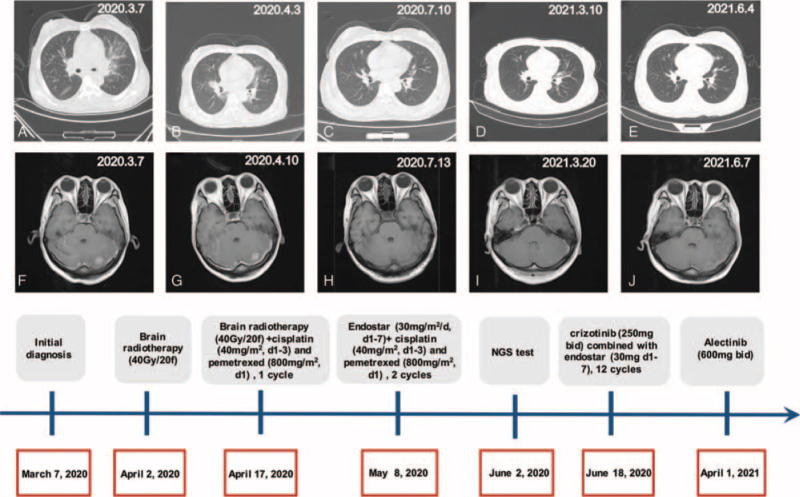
Chest computed tomography (CT) scan (A-E), magnetic resonance imaging (MRI) of the brain (F-J) and timeline of treatment. (A) CT of the lungs during the first physical examination. (B) Lung CT before receiving crizotinib treatment. (C) Lung CT after receiving crizotinib treatment. (D) Lung CT before receiving aletinib. (E) CT of the lungs after receiving aletinib. (F) Brain MRI of the first physical examination. (G) Brain MRI before crizotinib treatment (H) Brain MRI after crizotinib treatment. (I) Brain MRI before receiving aletinib. (J) Brain MRI after receiving aletinib.
